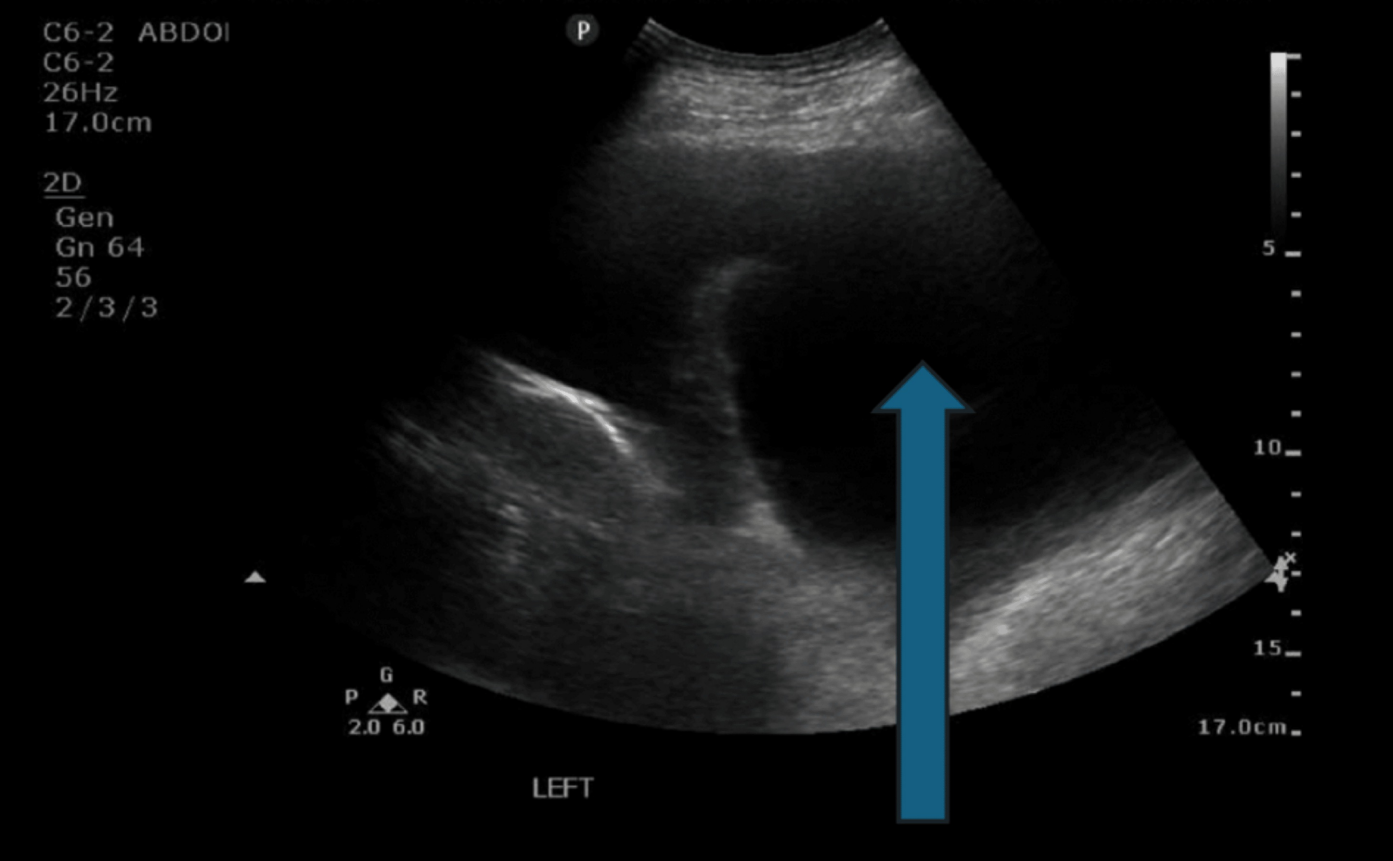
Left pleural effusion evident on ultrasound-guided thoracentesis in November 2024
